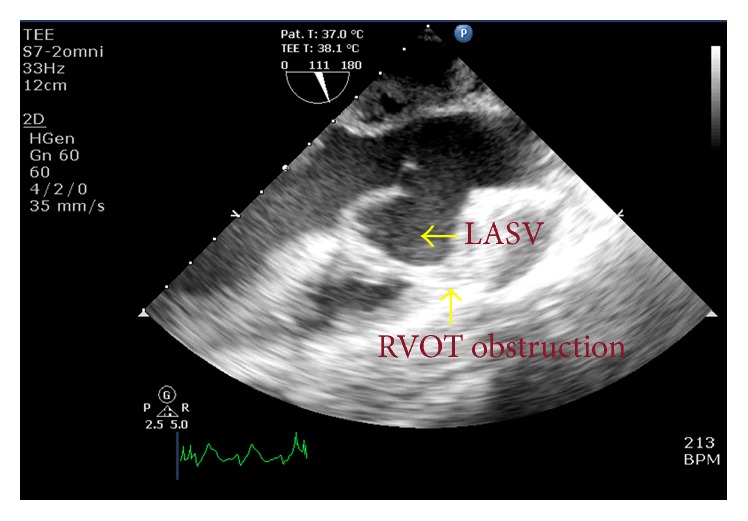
Transesophageal echocardiography^*∗*^ revealed left sinus of Valsalva aneurysm with thrombus within it causing RVOT obstruction. ^*∗*^Aortic valve long-axis view at midesophageal level.
